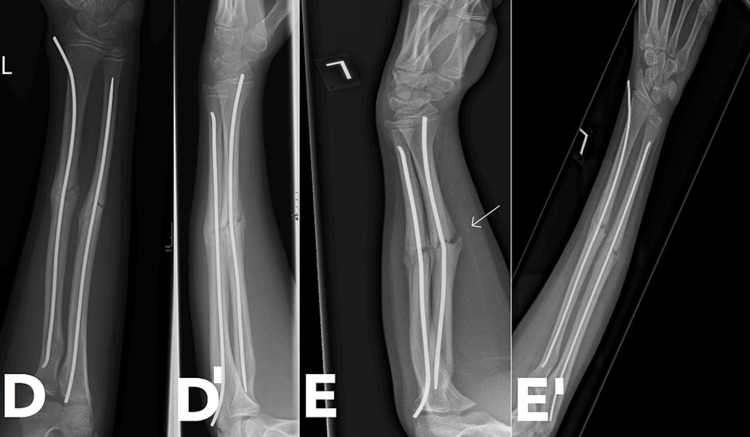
Follow up radiographs showing the refracture (D) AP view before refracture, note callus bridging the fracture; (D') Lateral view before refracture, note callus bridging the fracture; (E) Lateral view showing refracture, right arrow points to break in callus and bending of TENS in situ; (E') AP view showing refracture, no significant displacement in this view TENS: Titanium elastic nailing system
